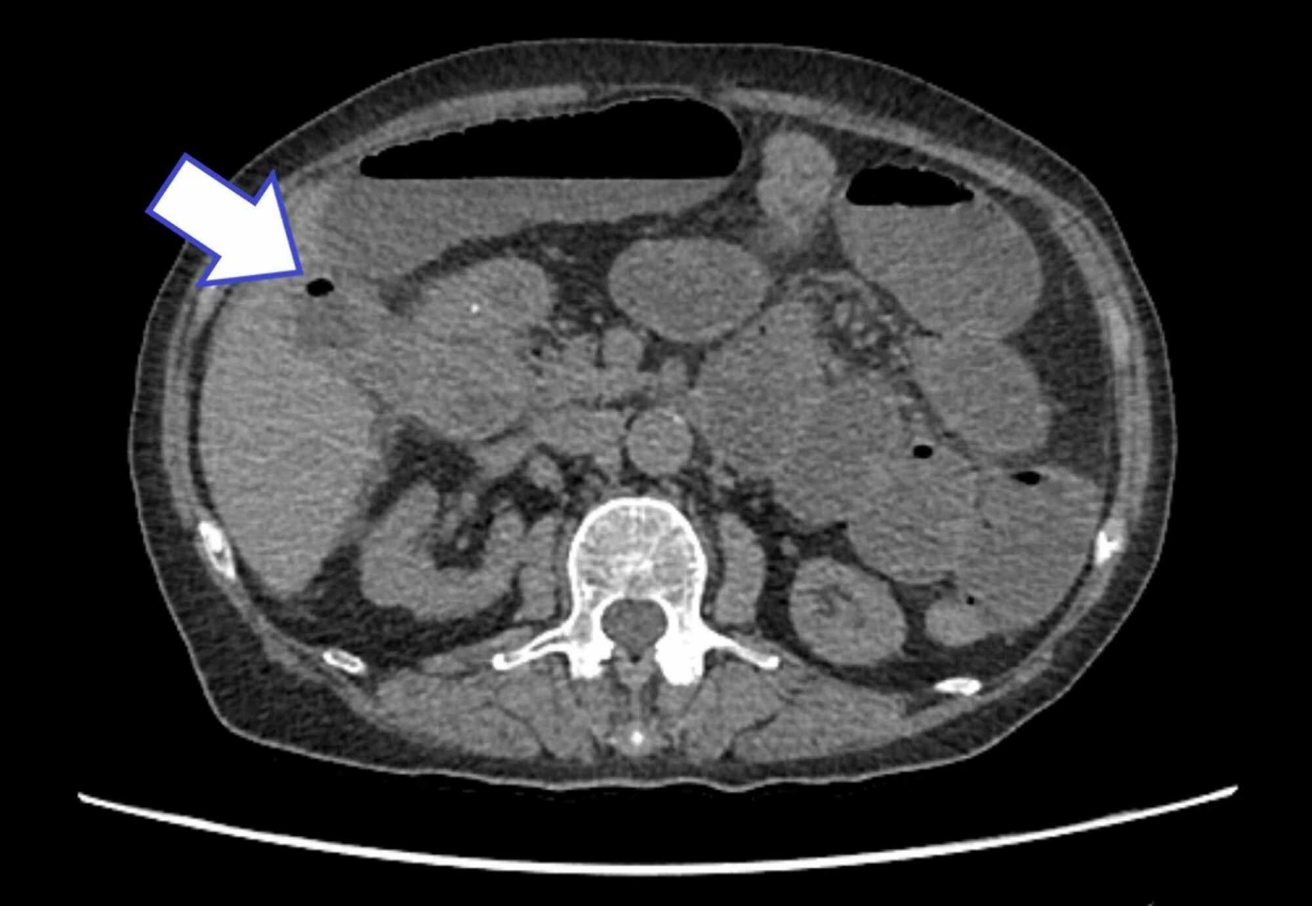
An axial cut CT scan showing air in the gallbladder fundus (white arrow indicates air within the gallbladder)
